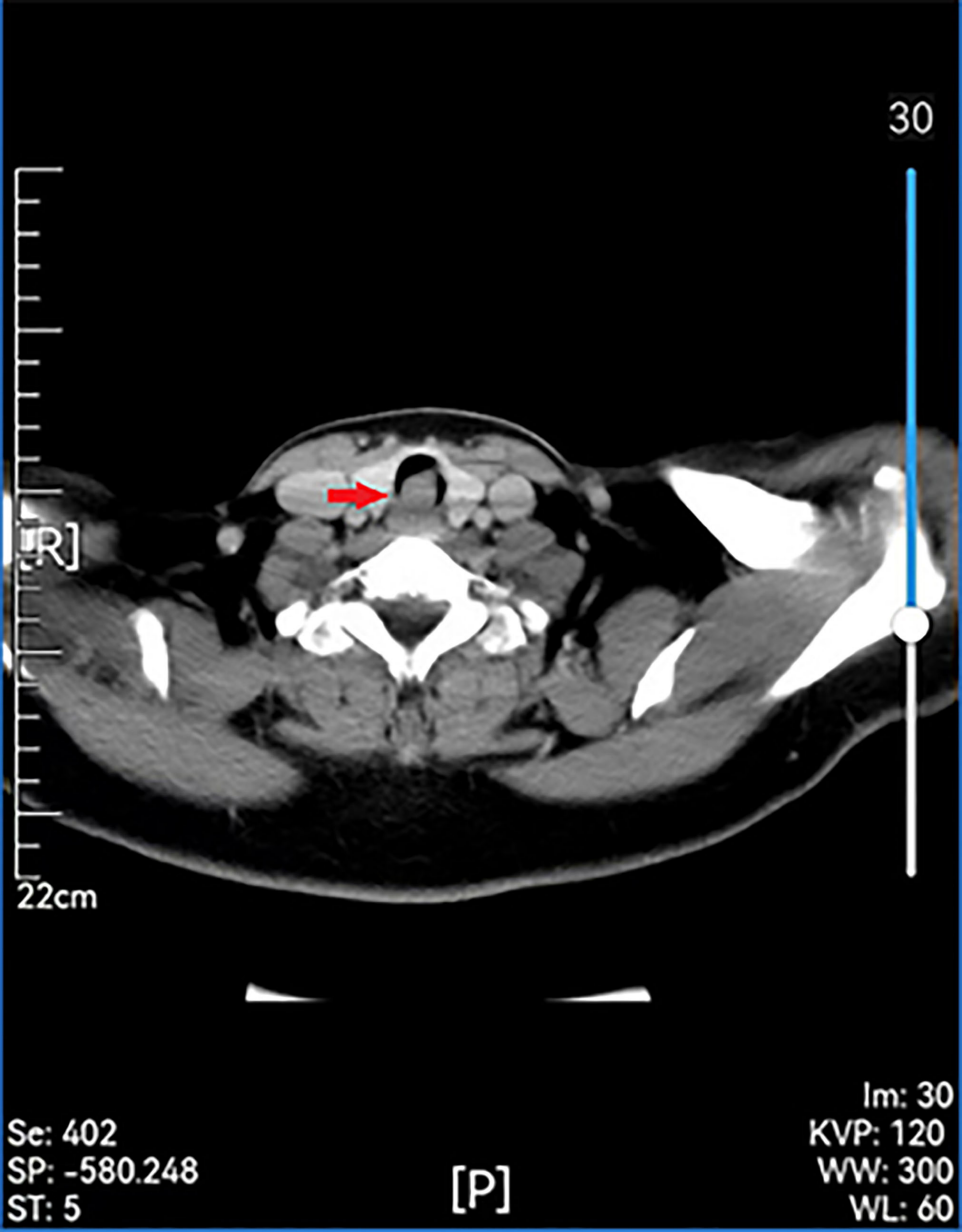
Preoperative enhanced CT scan showing a remodeled area of cervical with posterior wall erosion (red arrow).
